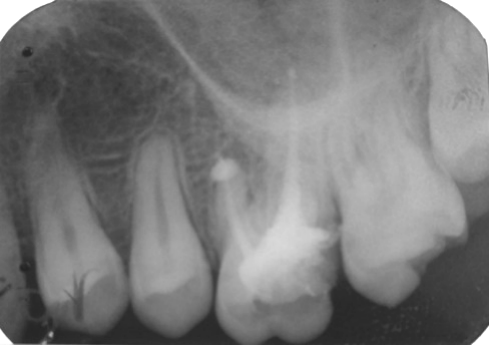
A periapical restriction radiolucent area on mesial, disto-vestibular and furcation level was observed on 2.6
